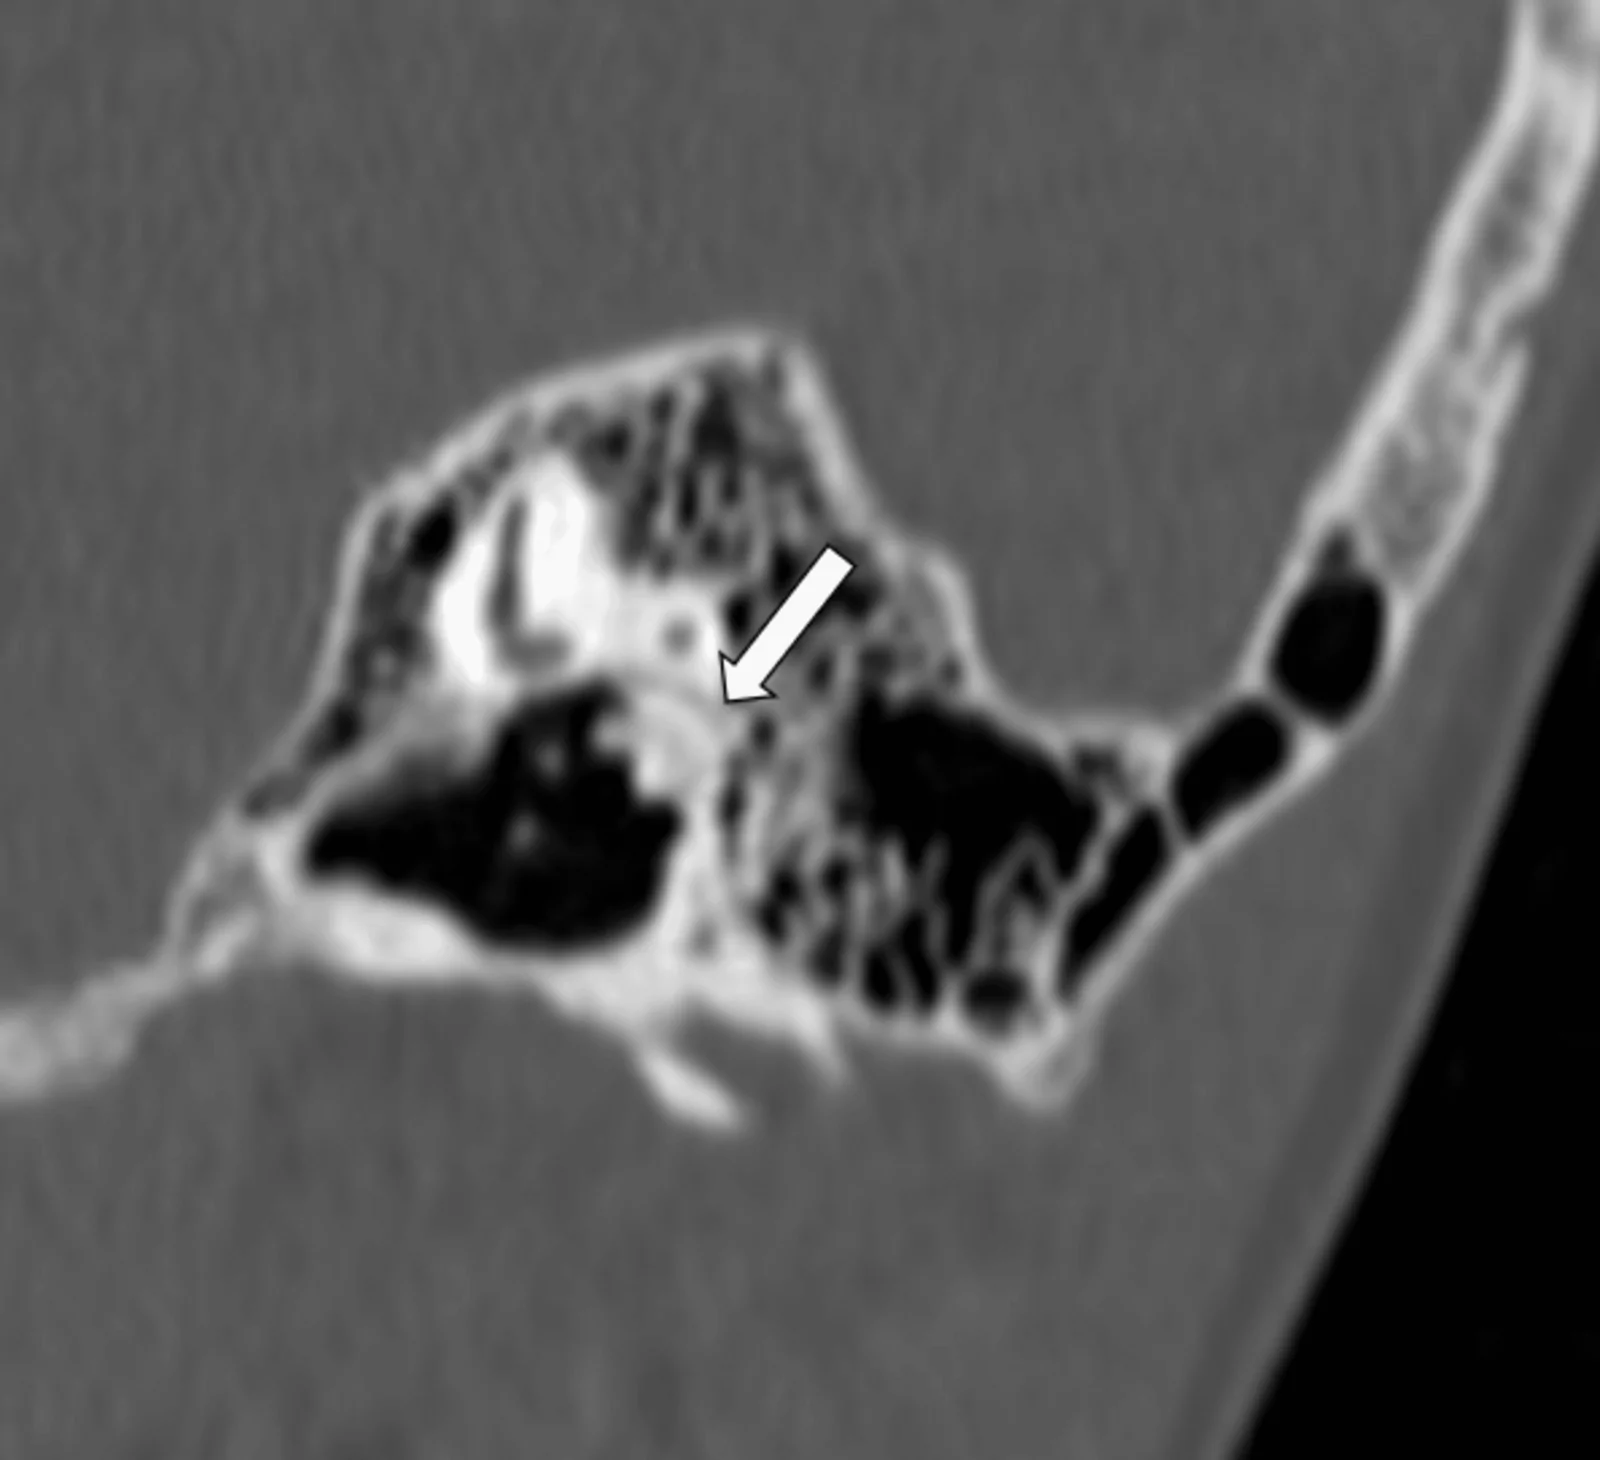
High-resolution computed tomography (HRCT) of the right temporal bone - oblique sagittal section The images demonstrate severe hypoplasia of the tympanic and aplasia of the mastoid segments of the facial canal. Straight arrow: severe hypoplasia of the tympanic part of the facial nerve canal Note that there is complete aplasia of the mastoid part of the facial nerve canal.
